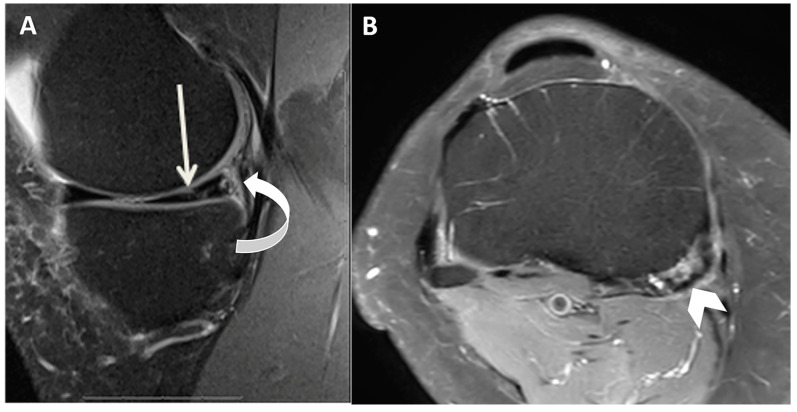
MRI of the right knee of a 31-year-old male patient who presented with medial-sided knee joint pain. Sagittal Proton Density Fat Saturated (A) and axial Proton Density Fat Saturated (B) MRI images demonstrate medial meniscal tear (arrow), posterior mensicocapsular separation (curved arrow), and semimembranosus tendinosis (chevron).
